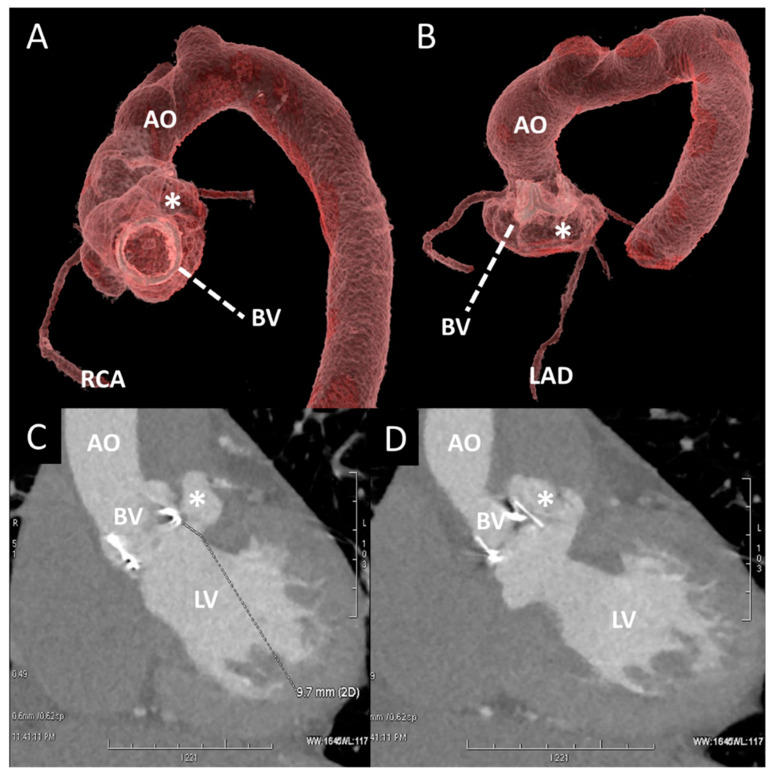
Contrast CT of a pseudoaneurysm (post endocarditis) of aortic root in a patient with aortic BV. (A,B) A 3D reconstruction of aortic root and thoracic aorta (AO). The asterisk shows the pseudoaneurysm adjacent to the left coronary sinus and the origin of left LAD. (C,D) Oblique planes of BV and aortic root: in (C), the white line shows the entrance of the pseudoaneurysm (*) right below the BV. BV, bioprosttic valve; LAD, left anterior descending artery; LV, left ventricle; RCA, right coronary artery.
